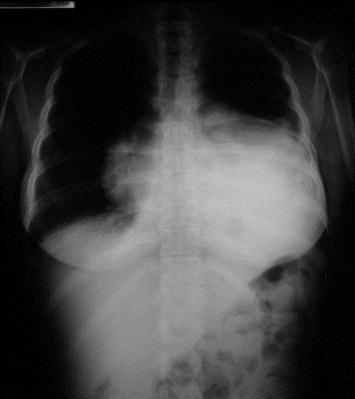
Preoperative chest x-ray demonstrating cardiac enlargement due to pericardial effusion.
